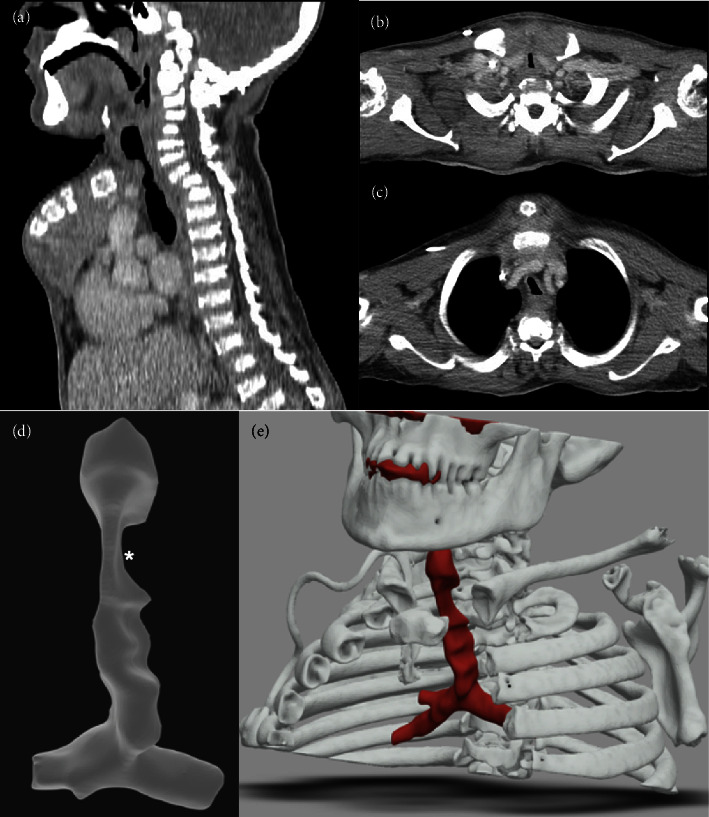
CT angiogram sagittal and (b) axial views immediately above and (c) below the manubrial head demonstrate multifactorial airway obstruction. Abnormal manubrium orientation and interposed brachiocephalic vasculature compressing the midtrachea into the c-spine, tracheal tortuosity, and tracheal ring deformity; shortened thorax with upper mediastinal compression; and cervico-thoracic kyphoscoliosis. (d-e) 3D-reconstructed images clearly demonstrate a tight thoracic inlet narrowing (^*∗*^).
